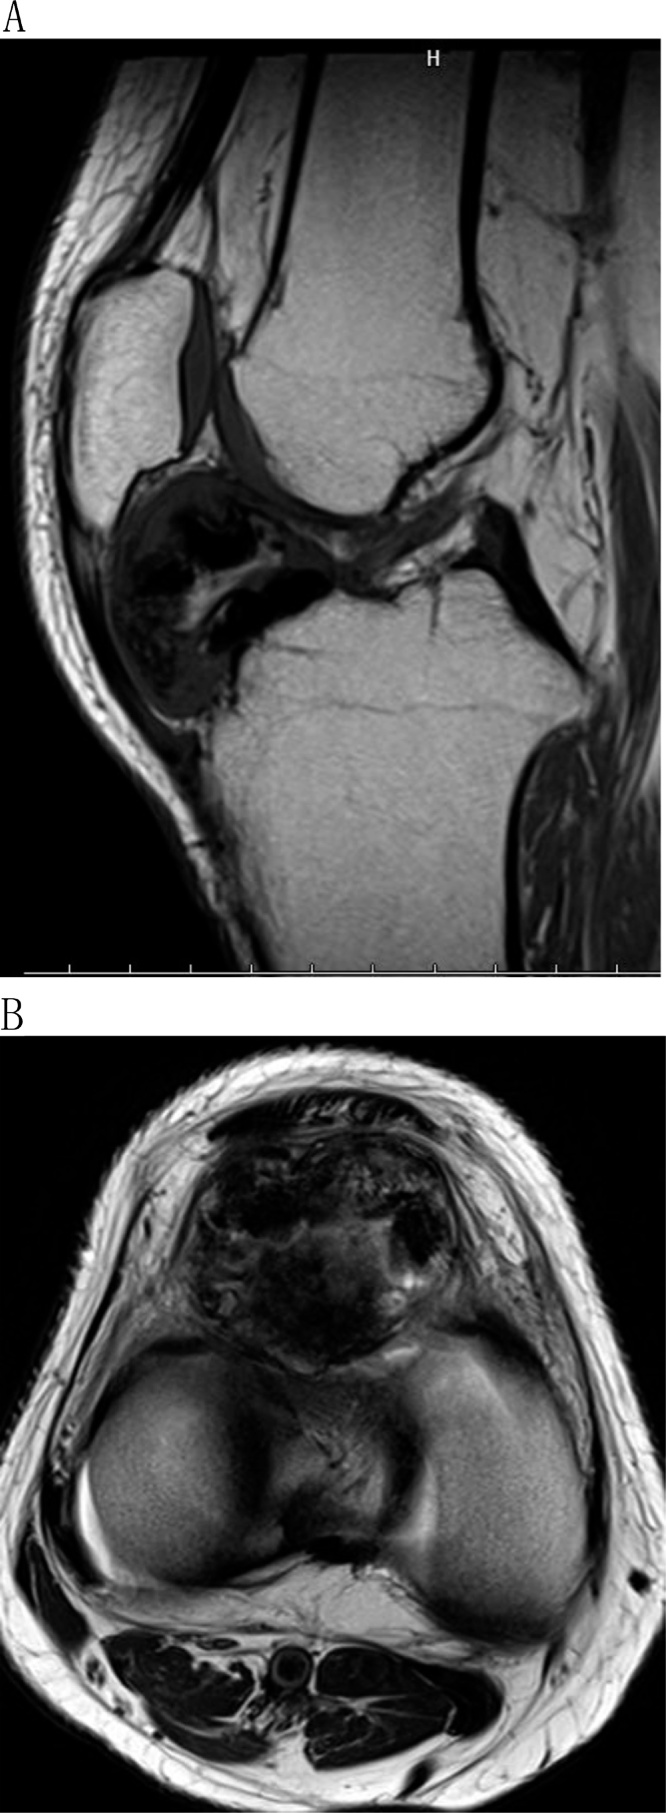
A: T1-weighted magnetic resonance imaging (MRI) showed low signal intensity in the infrapatellar fat pad that indicated ossification. B: T2-weighted MRI showed a high intensity area in the lesion that corresponded to cartilage.
